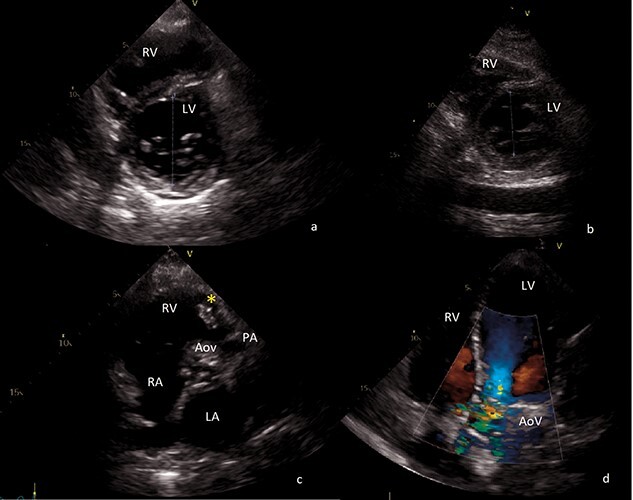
Transthoracic echocardiography images. (a) Preoperative short axis view of left ventricle. End-diastolic left ventricle diameter of 68 mm. (b) Postoperative short axis view of left ventricle. End-diastolic left ventricle diameter of 43 mm. (c) Sizable vegetation (yellow asterisk) of about 4 cm provoking right ventricular outflow obstruction. (d) Four-chamber view, showing severe aortic regurgitation. AoV aortic valve; LA left atrium; LV left ventricle; PA pulmonary artery; RA right atrium; RV right ventricle.
